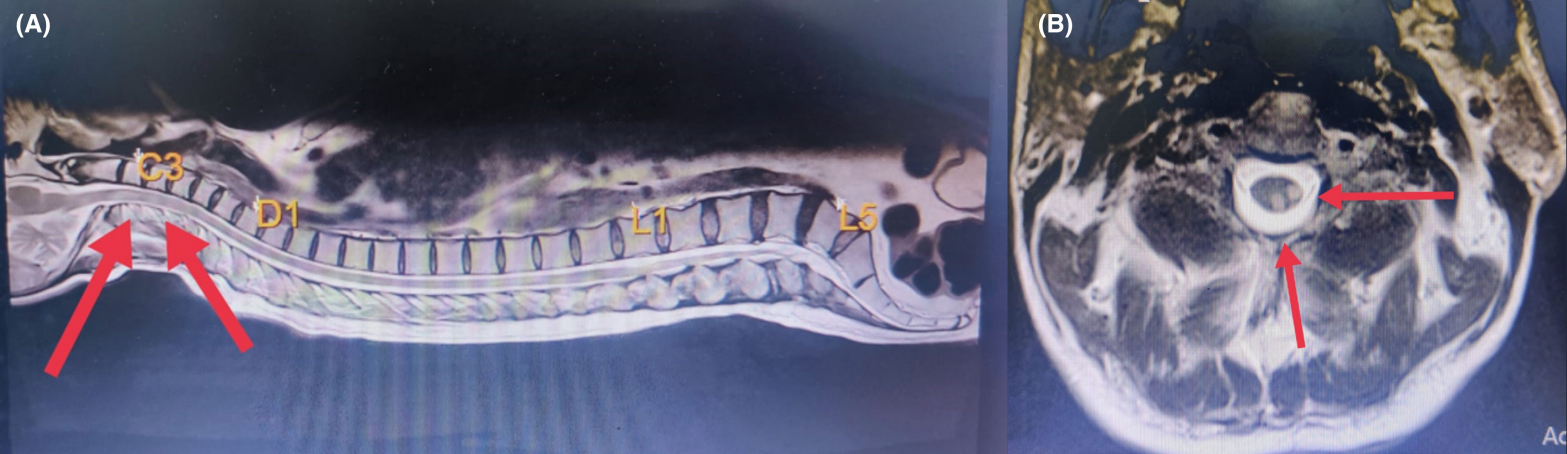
Transverse (A) and sagittal views (B) on spinal magnetic resonance imaging suggest acute transverse myelitis involving C3‐4.
